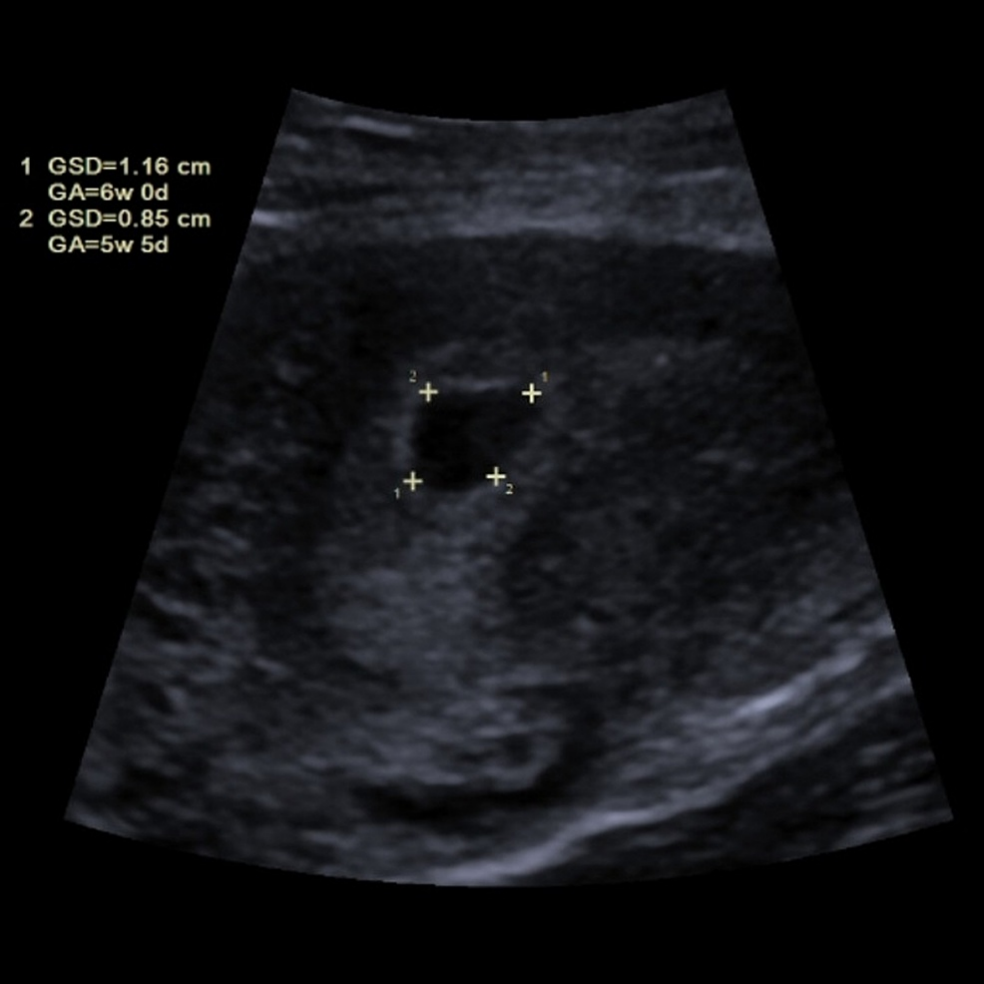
Transabdominal ultrasound showing the gestational sac.
